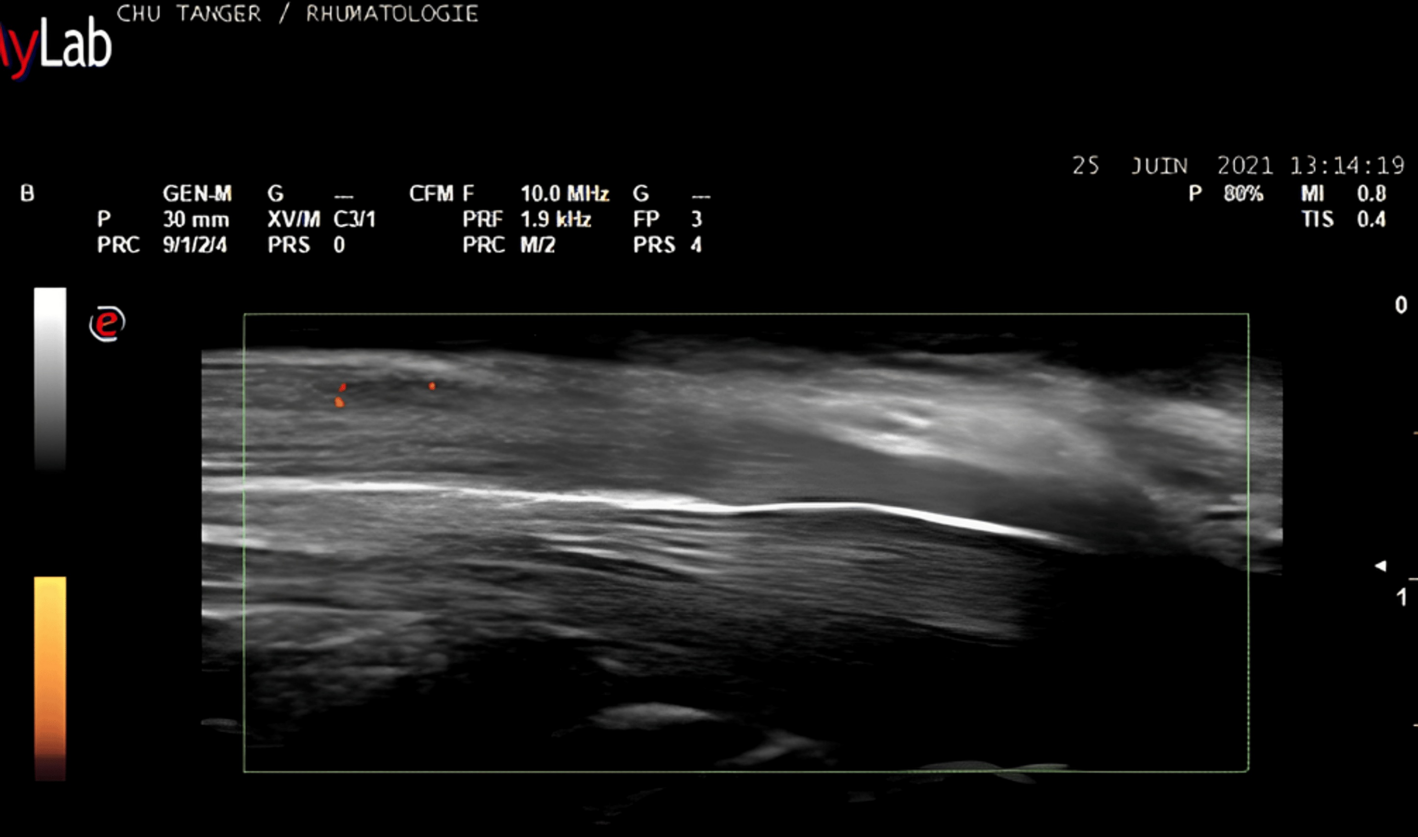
A longitudinal ultrasound image demonstrates intraneural vascularisation of the median nerve.
